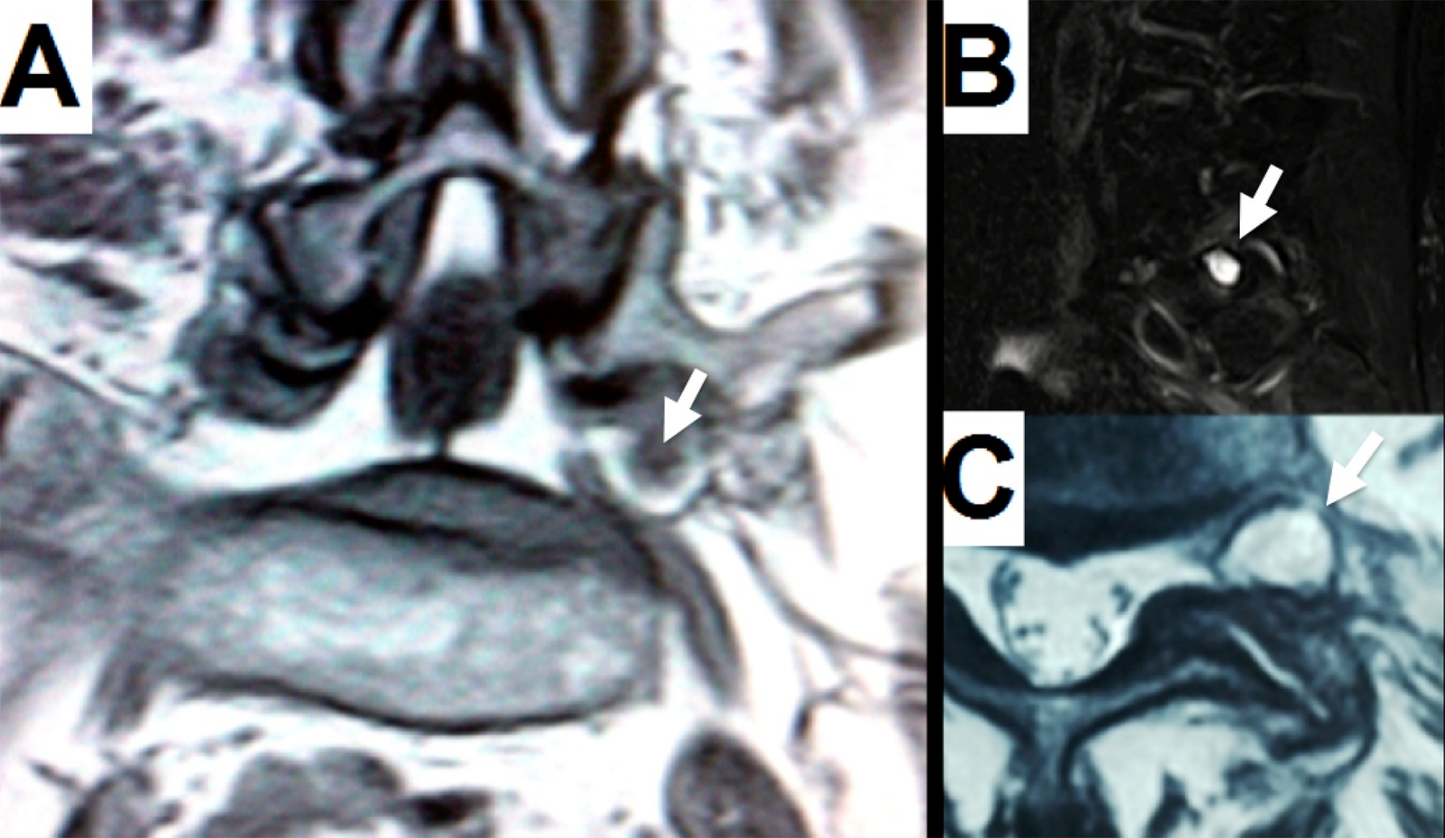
Preoperative MRI A: Coronal T2 shows a hyperintense cyst caudally displacing the root and compressing it with the sacral wing. Black content inside the cyst represents previous bleeding (white arrow). B: Sagittal T2 displays a hyperintense cyst on the left extraforaminal space (white arrow). C: Axial T2 shows hypertrophy of the facet joint into the left extraforaminal space (white arrow).
